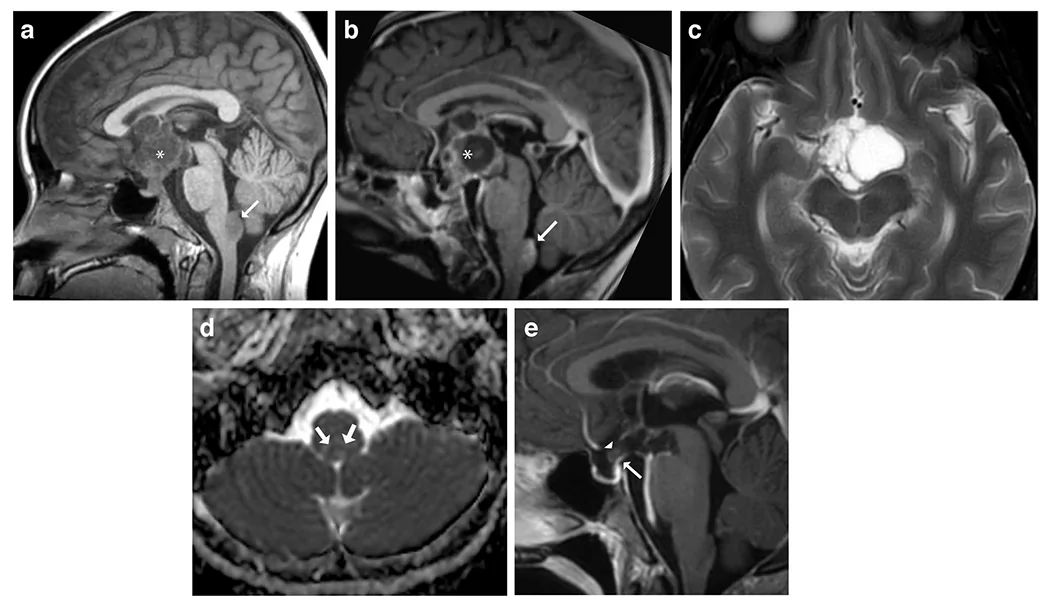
Suprasellar germinoma and metastatic focus in a 15-year-old female with primary amenorrhea and altered mental status at initial presentation. Tumor resection resulted in central diabetes insipidus, central hypothyroidism, and adrenal insufficiency. Sagittal (a) pre-contrast and (b) post-contrast T1-weighted image through the midline shows a large multilobulated, heterogeneous mixed cystic and solid mass with enhancement of solid components (*asterisk*) and hypointense ill-defined and faintly enhancing lesion at the cervicomedullary junction (*arrow*). Axial (c) T2-weighted image demonstrates multiple non-enhancing cystic components intermixed with enhancing solid tissue. d Axial ADC shows a rim of low-apparent diffusion coefficient (*arrows*, isointense to cerebellum) of the round metastatic focus at the obex. e Sagittal post-contrast T1-weighted image through the midline at 1-year follow-up surveillance exam shows post-resection findings. The sella has an empty appearance. Pituitary infundibulum is visualized (*arrow*), and enhancing tissue along floor of sella suggests a thin rim of residual adenohypophysis. Residual nodule of non-enhancing tissue (*arrowhead*) at the level of the hypothalamus remained stable over subsequent exams (not shown)
